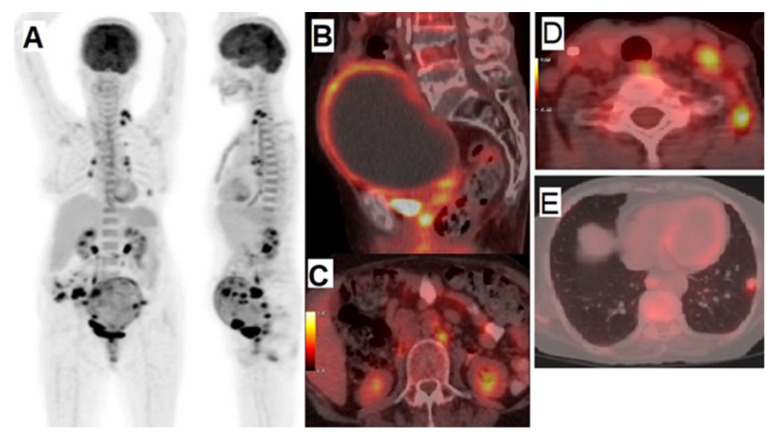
A 66-year-old female presented with a pelvic mass and vaginal bleeding. Cervical squamous cell carcinoma (SCC) was confirmed via biopsy and referred for staging. (A) Disease distribution visualized with [^18^F]FDG PET/CT imaging. (B) Sagittal pelvis image showing primary tumor leading to hydrometra and regional lymphadenopathies. (C) Retroperitoneal and (D) Supraclavicular lymph node metastases, in addition to lung metastases (E). (Pictures with courtesy from Department of Nuclear Medicine, Vali-Asr Hospital, Tehran University of Medical Sciences, Tehran, Iran).
